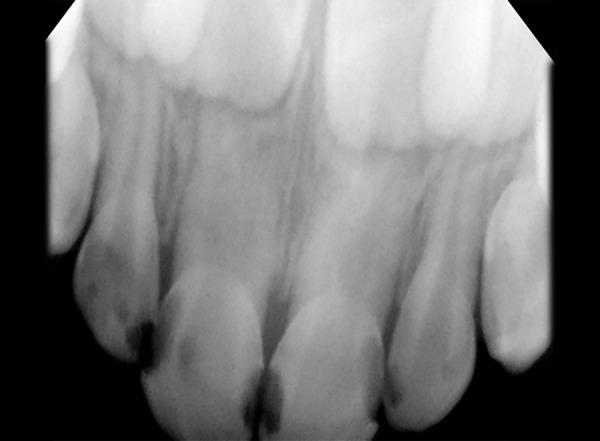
Intraoral periapical view with pulpal involvement with 51, 52, 61, 62
